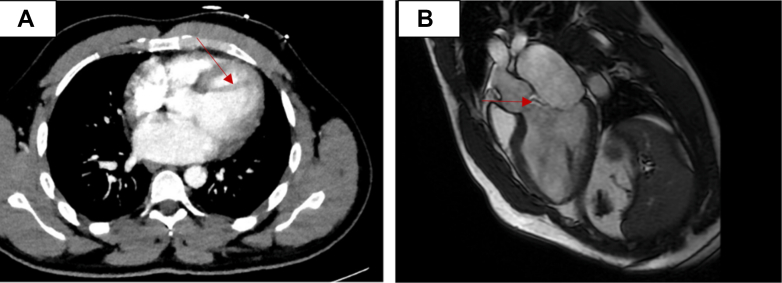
Multimodal Imaging (A) Computed tomography scan of the pulmonary arteries with intravenous contrast demonstrates a hypointense structure in the left ventricular outflow tract (red arrow). (B) Cardiac magnetic resonance scan demonstrates a mobile linear hypointense structure (red arrow) extending inferiorly from the left coronary cusp into the LVOT, which approaches the A1 scallop of the mitral valve.
